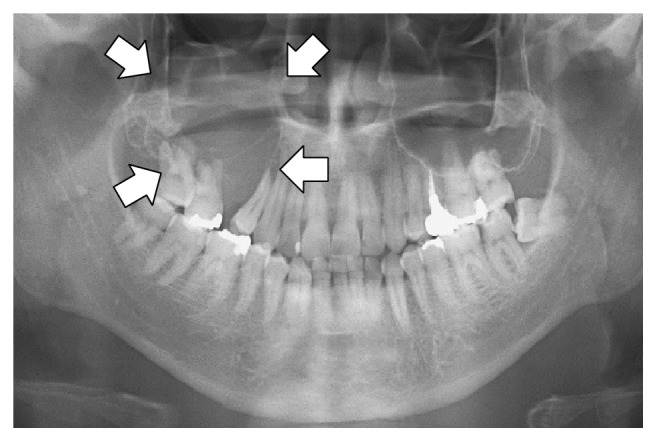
Panoramic radiography shows an osteolytic lesion in the right posterior maxilla with resorption of the floor of the maxillary sinus (arrows).
